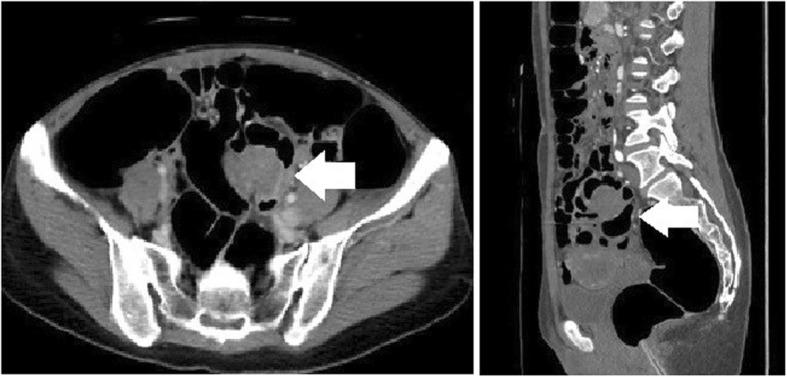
Computed tomography shows the mass in the sigmoid colon (arrow)
